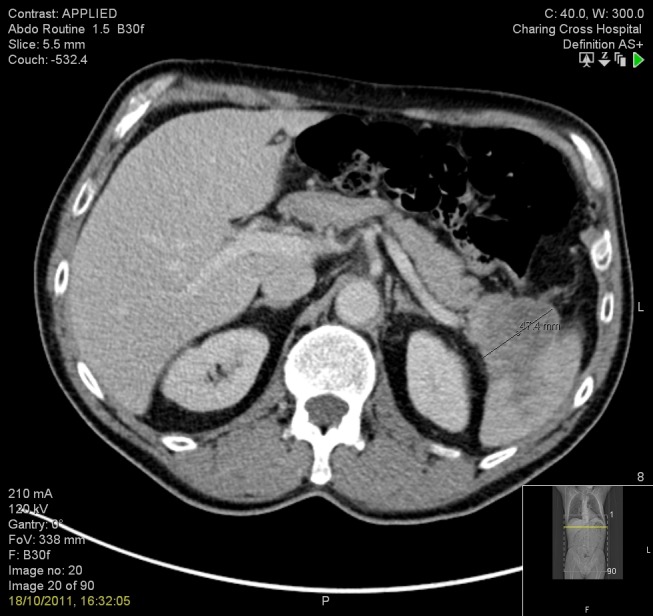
CT showing primary pancreatic tumour
